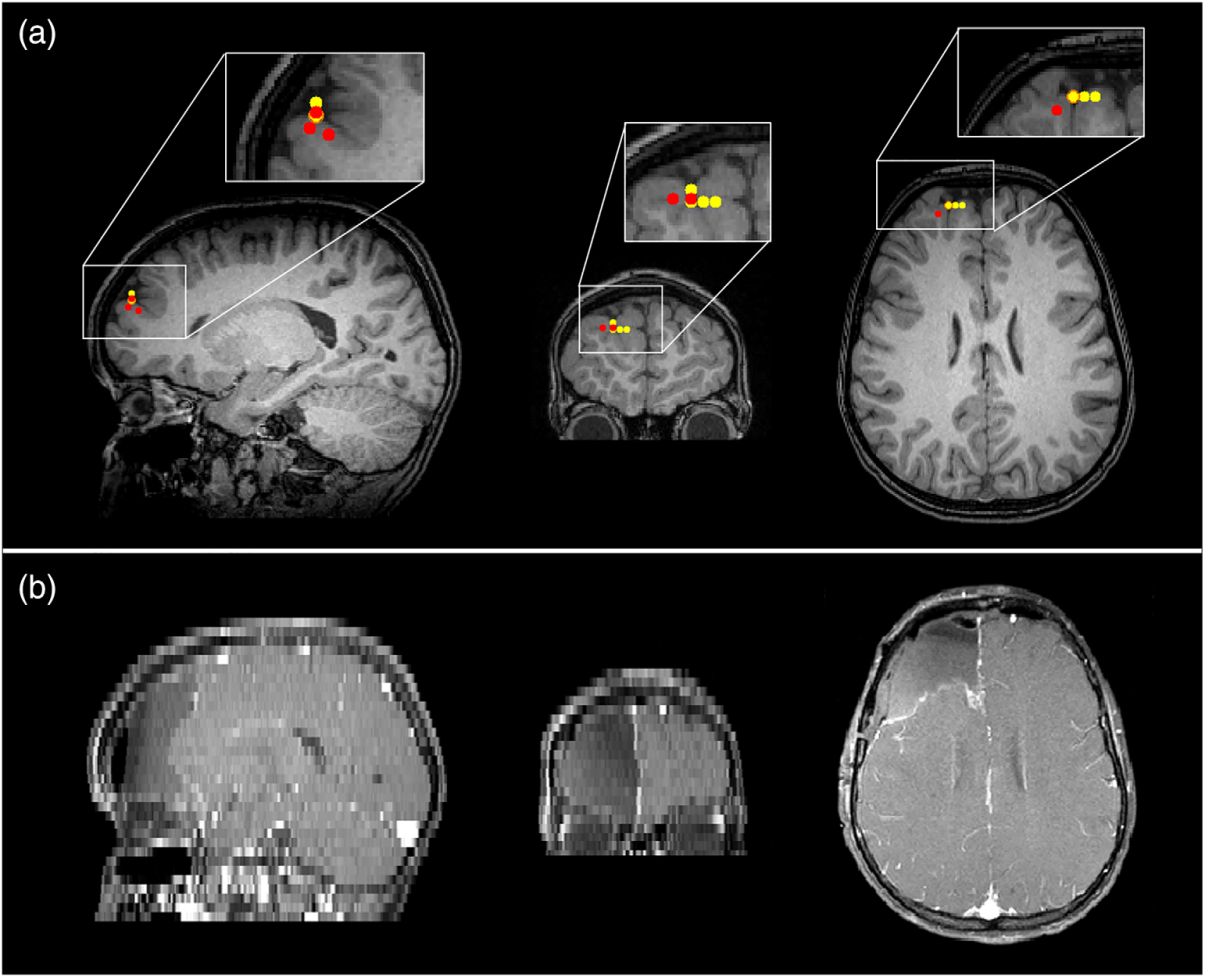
Epilepsy Case 1—magnetoencephalography (MEG) localizations compared with resected area for patient 2 (focal spike wave). Panel (a) The MEG localizations for the excess kurtosis mapping method (red markers) and the hidden Markov model method (yellow markers). Panel (b) shows the resected area in the postoperative magnetic resonance imaging (MRI). Note that the postoperative MRI has different resolutions in the sagittal, coronal, and axial planes
